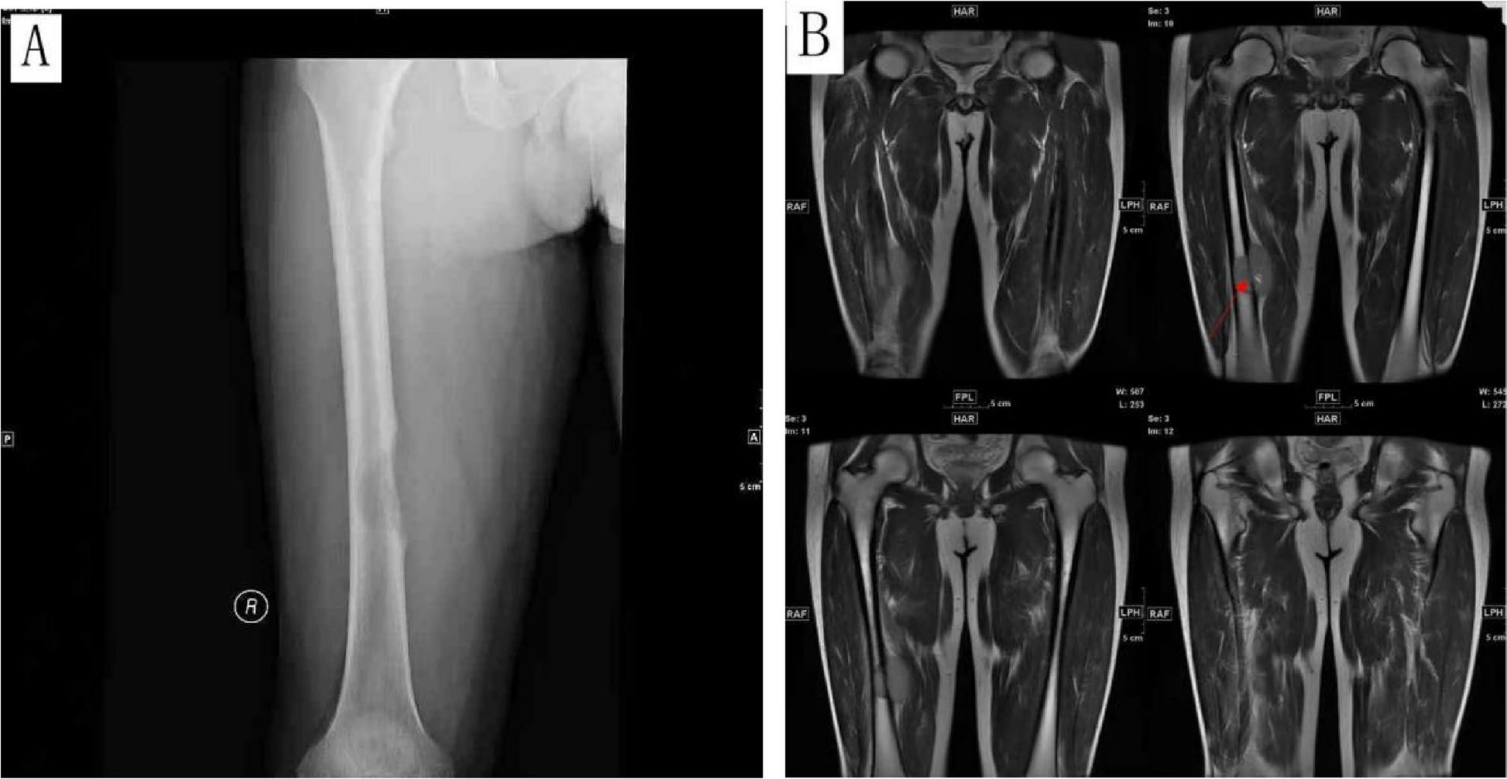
Case 1: Radiograph (A) and MRI (B) of the right femoral shaft tumor show lytic lesions involving the bone and surrounding soft tissues
